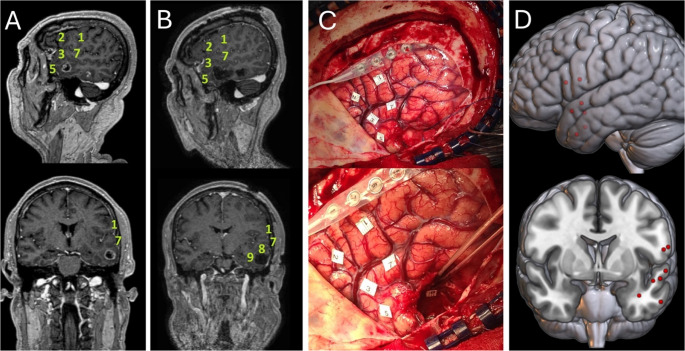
Preoperative (A) and postoperative (B) MRI scans of a patient affected by left temporal glioblastoma (GBM). Numbers on the cortical and subcortical surfaces were positioned by comparing the MRI with an intraoperative picture of the labeled brain after mapping (C). In (D), these points are transposed onto the 3D Montreal Neurological Institute (MNI) brain template
